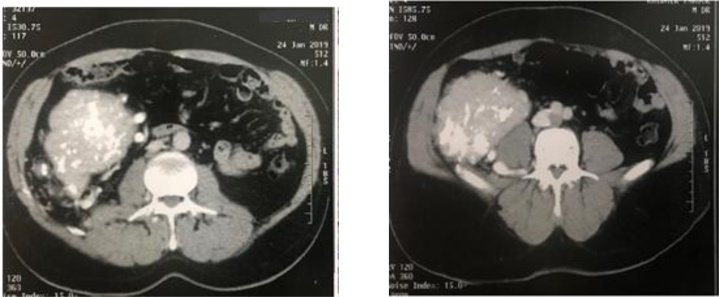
CT scan of the abdomen shows a well-defined enhancing mass.
